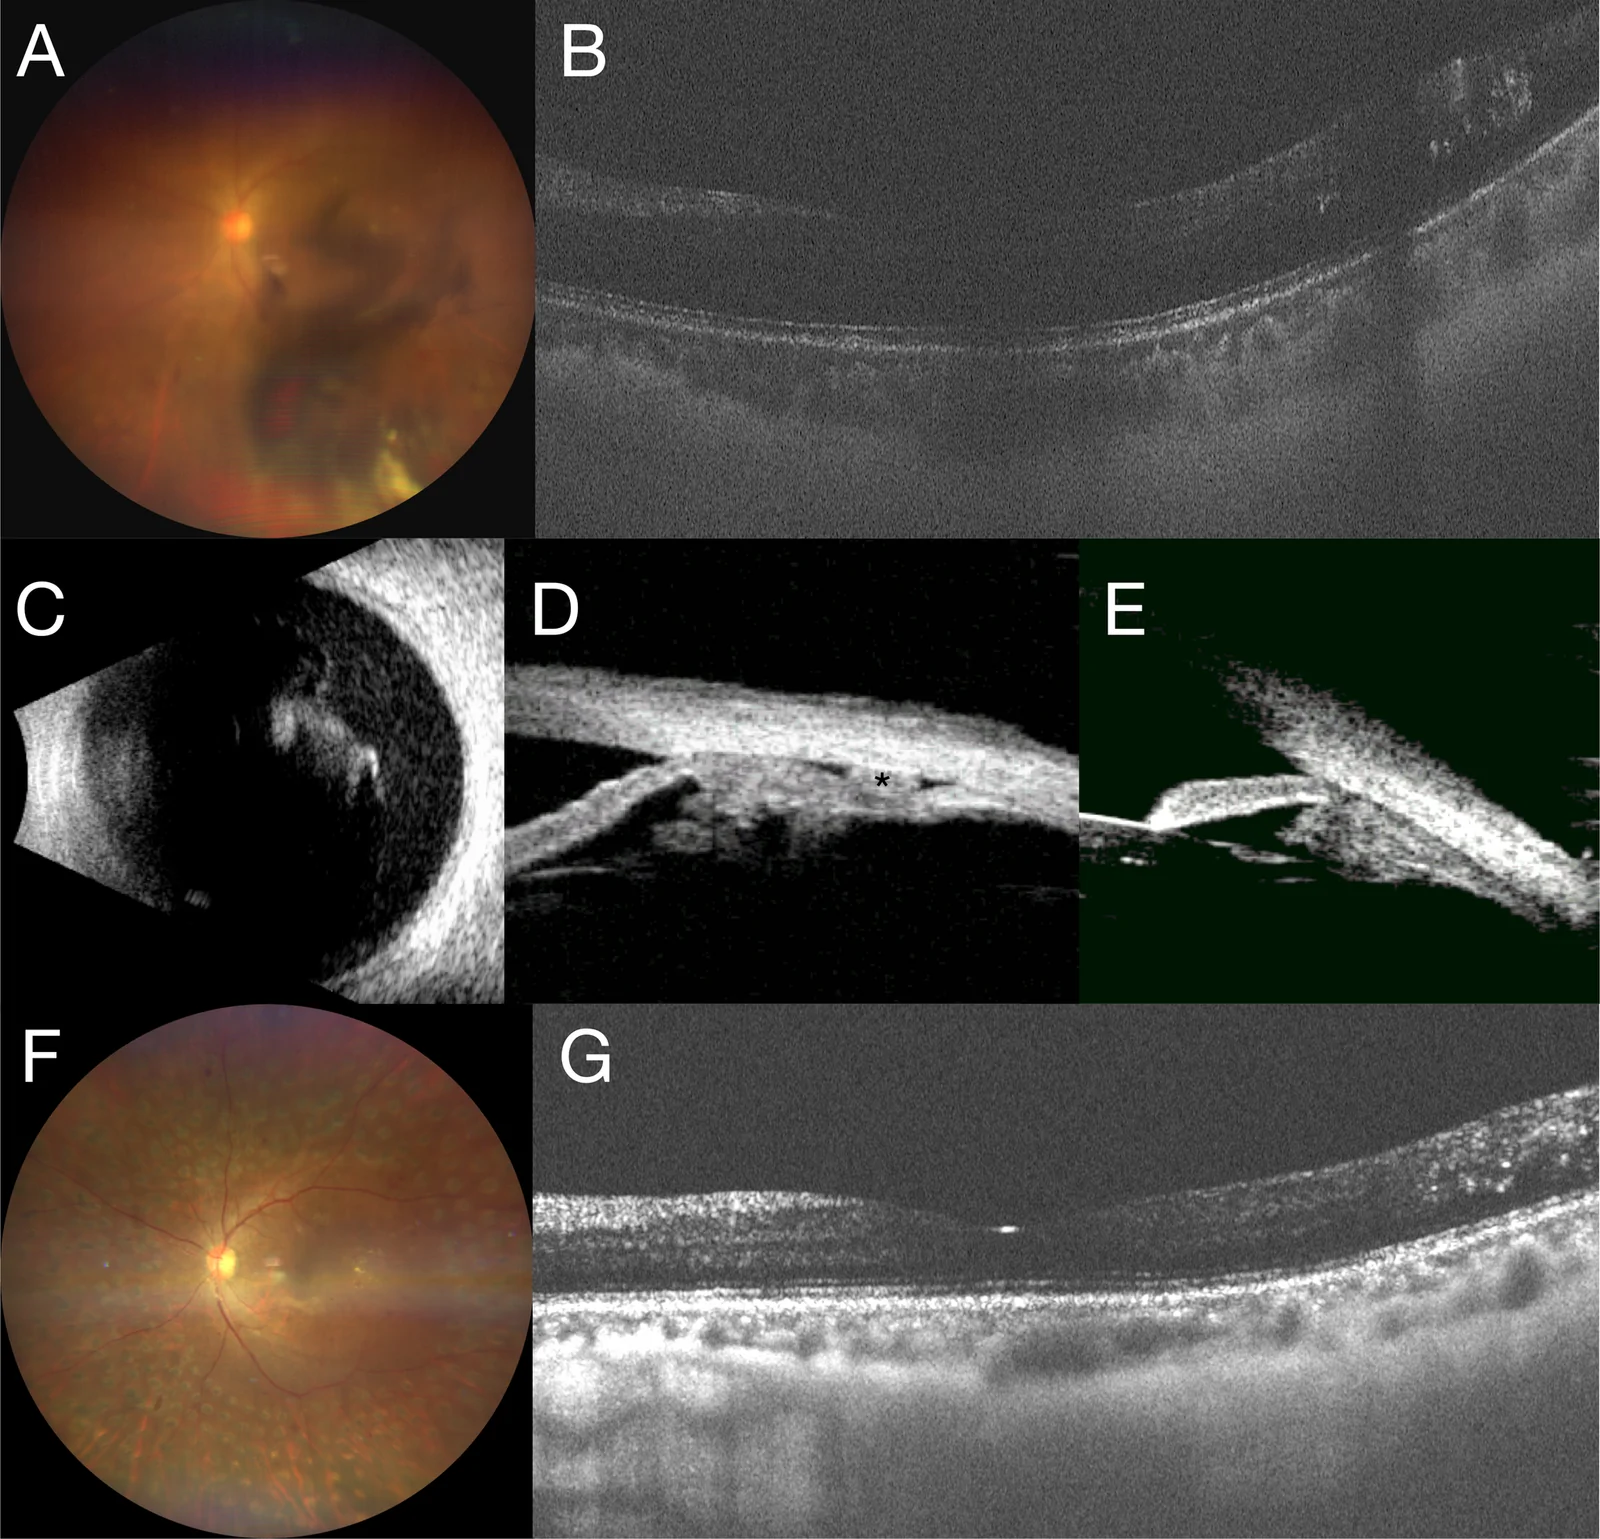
Pre- and post-operative clinical presentations of the patient’s left eye. A, fundus photography of the patient’s left eye, showing vitreous hemorrhage obscuring view of retina and peripheral post photocoagulation changes. B, OCT of left eye, revealing intraretinal cysts and hyperreflectivity. C, B-scan ultrasonography of left eye, revealing clumped and strand-like echoes in the vitreous cavity. D, UBM of left eye a week after surgery, revealing anechoic spaces between the pars plana and the sclera (12:00 position). Asterisk indicated the injected suprachoroidal Ozurdex. E, UBM of left eye 12 weeks after surgery, revealing the disappearance of this anechoic space, indicating the absorption of Ozurdex injected to SCS. F, fundus photography of left eye at 12-week follow-up, showing a clear view of retina in both eyes with few exudates and peripheral post photocoagulation changes. G, OCT of left eye at 12-week follow-up, revealing resolved intraretinal cyst but persistent intraretinal hyperreflectivity
